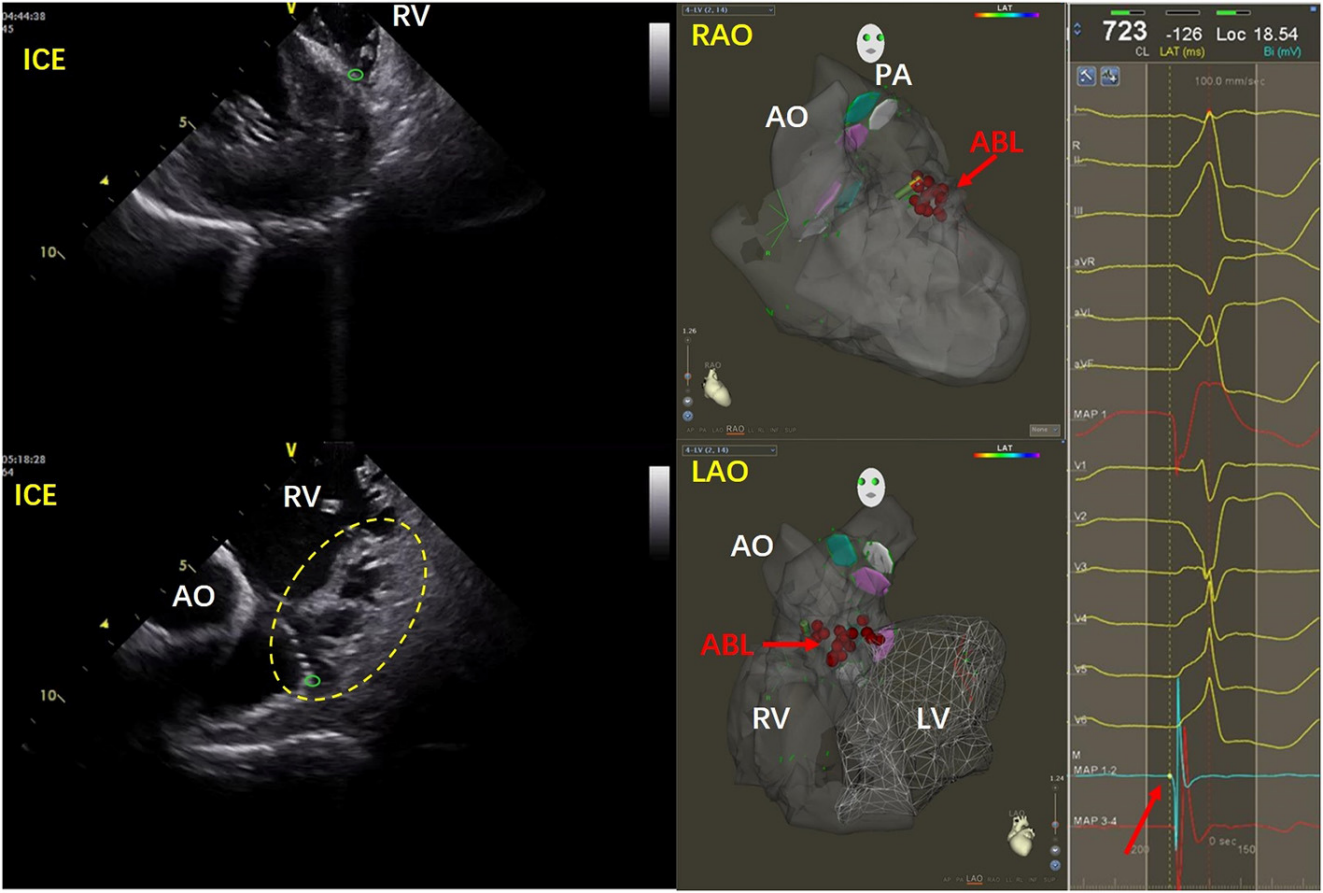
Images for a patient with PVCs that originated from the inferoanterior septum in the inferior RVOT. In the ICE image on the left, the tip of the ablation catheter is indicated by the green circle. Abundant trabeculae can be seen in the yellow dotted line area. The tip of the ablation catheter is visible embedded at the intersection of the muscle trabeculae at their junction with the smooth conus of the pulmonary artery. The red arrow in the middle three-dimensional image shows the ablation target. On the right side of the figure, the red arrow indicates the early potential at the ablation site. ABL, ablation; AO, aorta; ICE, intracardiac echocardiography; LAO, left anterior oblique; LV, left ventricle; PA, pulmonary artery; RAO, right anterior oblique; RV, right ventricle.
